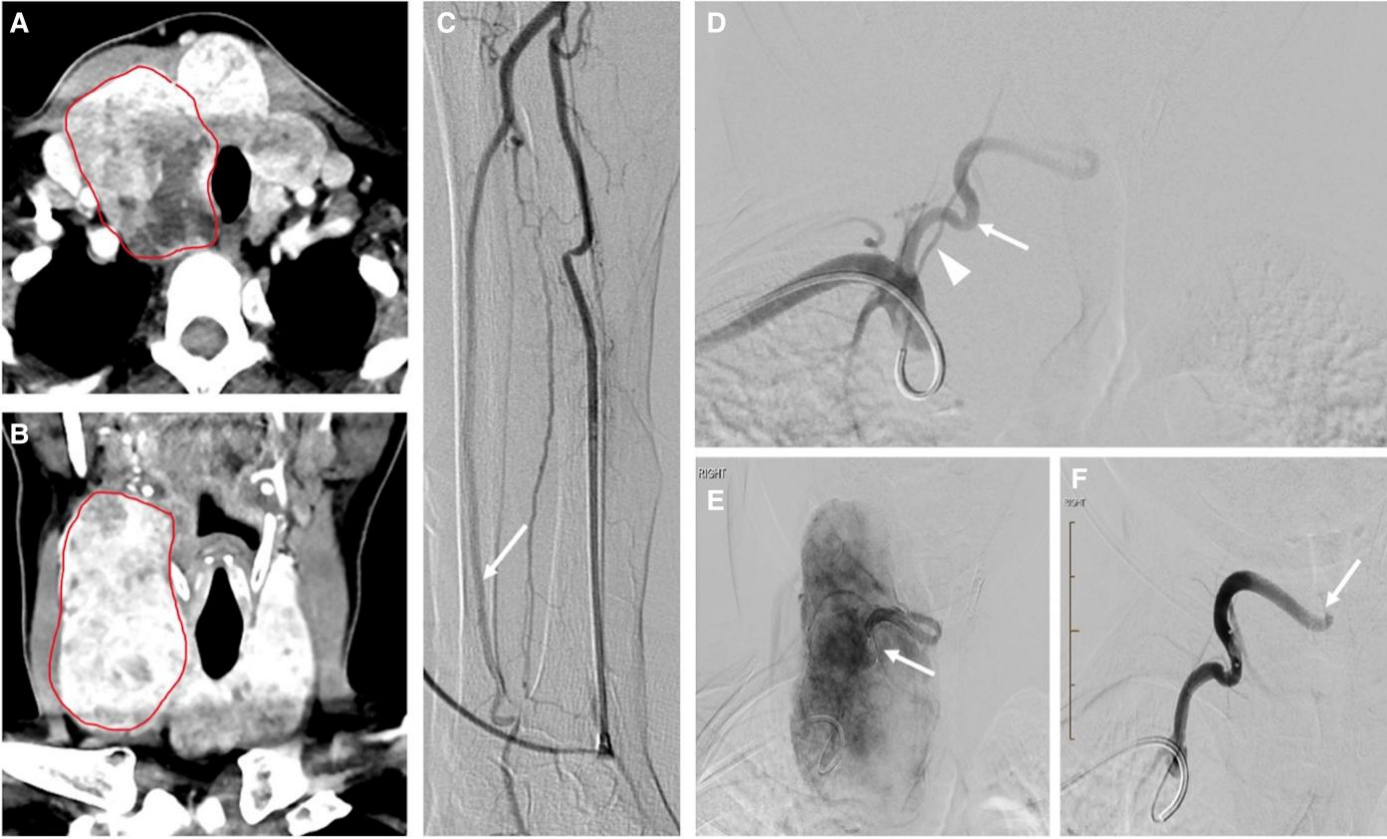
The pressure-enabled thyroid embolization technique. A, Computed tomography (CT) of the neck with contrast, axial view, demonstrating a multinodular goiter, predominantly involving the right lobe with contralateral tracheal displacement. The red circumference marks the target volume for embolization. B, CT of the neck with contrast, coronal view, demonstrating the same imaging findings. The red circumference marks the target volume for embolization. C, Right forearm angiogram demonstrates no significant radial loop and patency of the ulnar artery and palmar arch (arrow). D, Subclavian angiogram demonstrates a significantly enlarged inferior thyroid artery off the subclavian artery (arrow). Note the proximity of the ipsilateral vertebral artery (arrowhead). E, Angiography following catheterization of the right inferior thyroid artery with the pressure-enabled drug delivery device. The device is always positioned after the first turn of the artery, in its first descending segment (arrow). Note the significant goiter hypervascularity without arterial reflux. F, Flow stagnation within the right inferior thyroid artery following embolization with resolution of the parenchymal blush.
